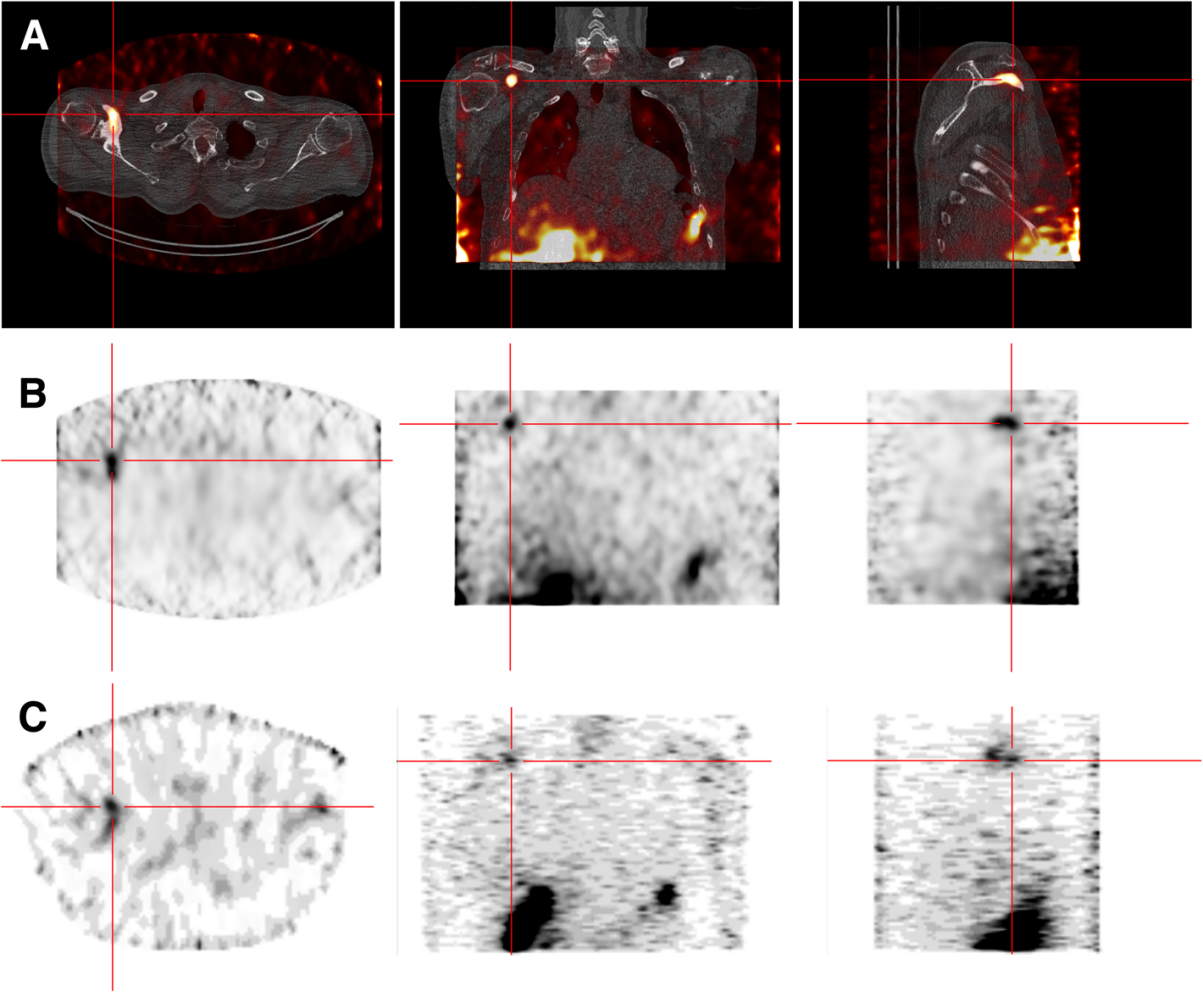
SPECT imaging of 30-min duration performed successively on CzT (with (a) and without (b) CT image fusion) and Anger cameras (c) 48-h after intravenous injection of 5.27 MBq of ^223^Ra. An uptake on a right shoulder bone metastasis was visible on axial, coronal and sagittal slices (left to right)
